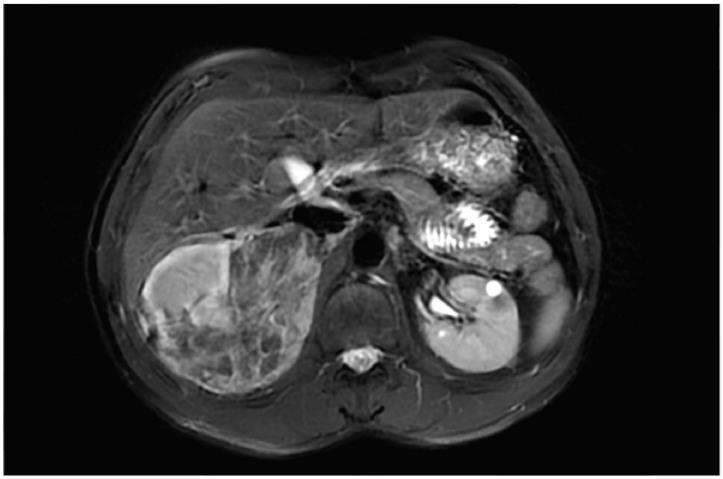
MRI of a male patient with tuberous sclerosis complexshowing a large angiomyolipoma in the right kidney and a cyst in the left kidney.
